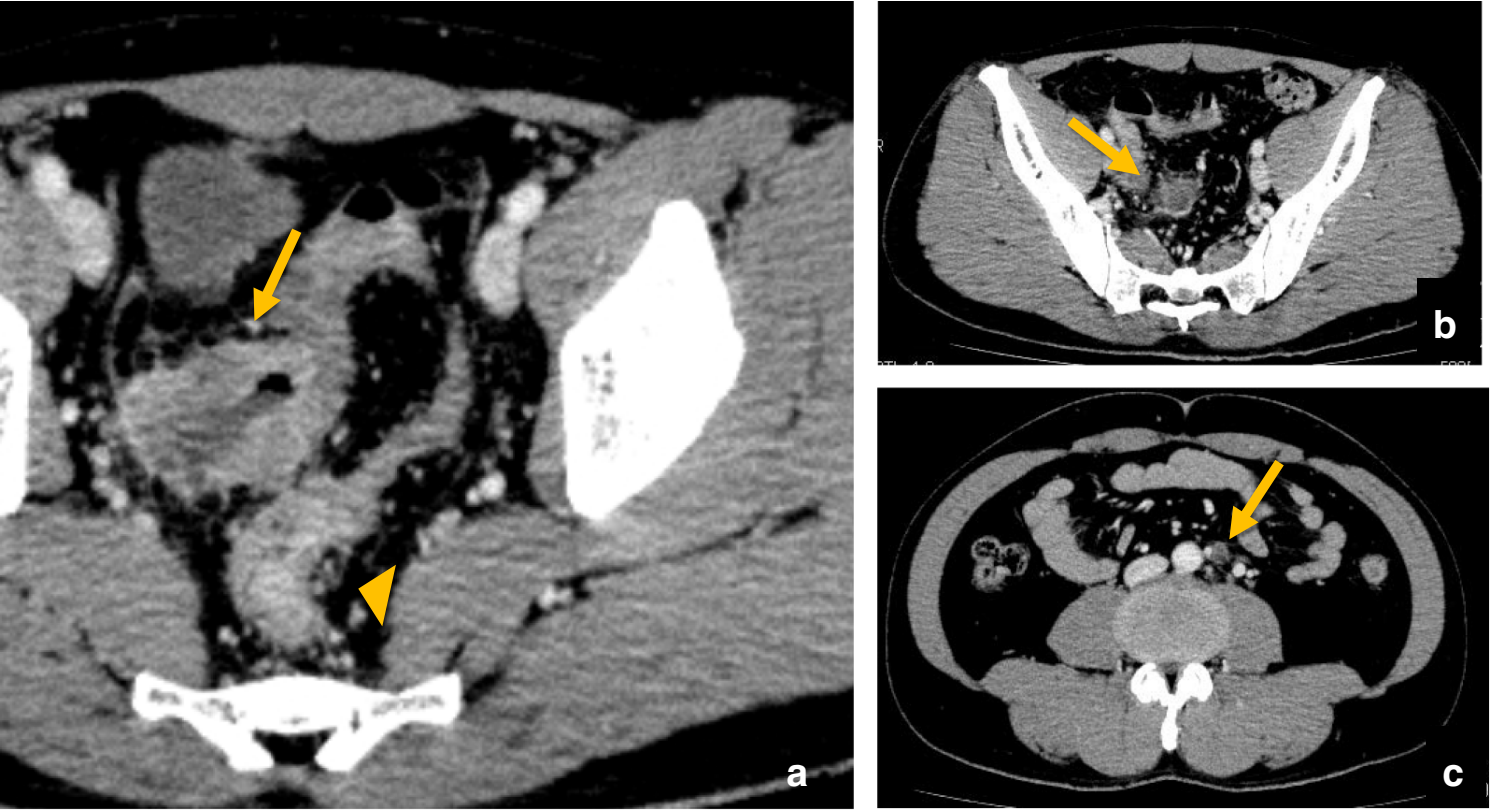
Computed tomography (CT) scan showed two wall-thickening lesions in the rectum (arrowhead) and sigmoid colon (arrow), with the latter invading the small intestine and abdominal wall (a). Lymph nodes were swollen in the sigmoid mesocolon (b) and at the roots of the inferior mesenteric artery (c). Some of the swollen lymph nodes had sac-like degeneration inside (b)
